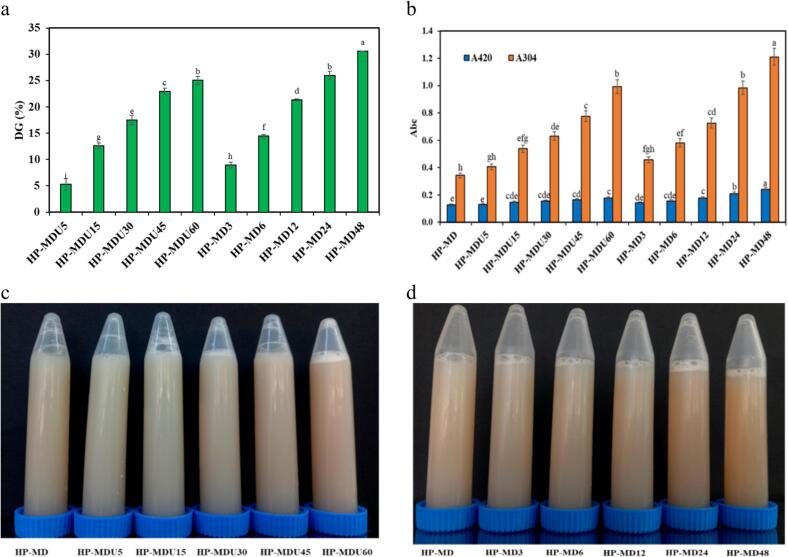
(a) Glycation degree% (DG) (b) absorbance at 420 and 304 nm of samples, (c) Visual alteration of ultrasound (d) and wet-heating treatments.
